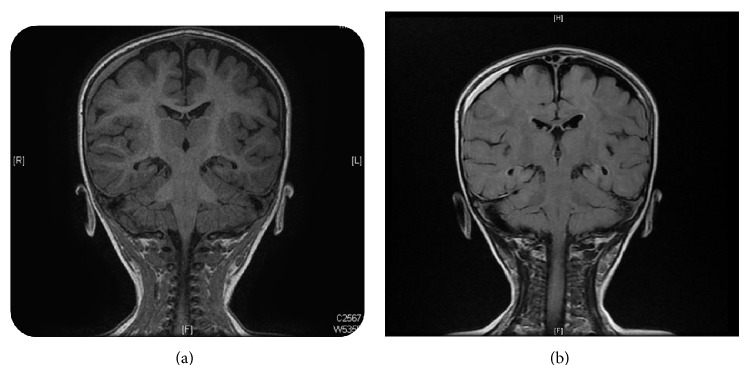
Brain magnetic resonance imaging (MRI) showed cortical and cerebellar atrophy, right-sided subdural collection noted over the right frontal and parietal convexity.
